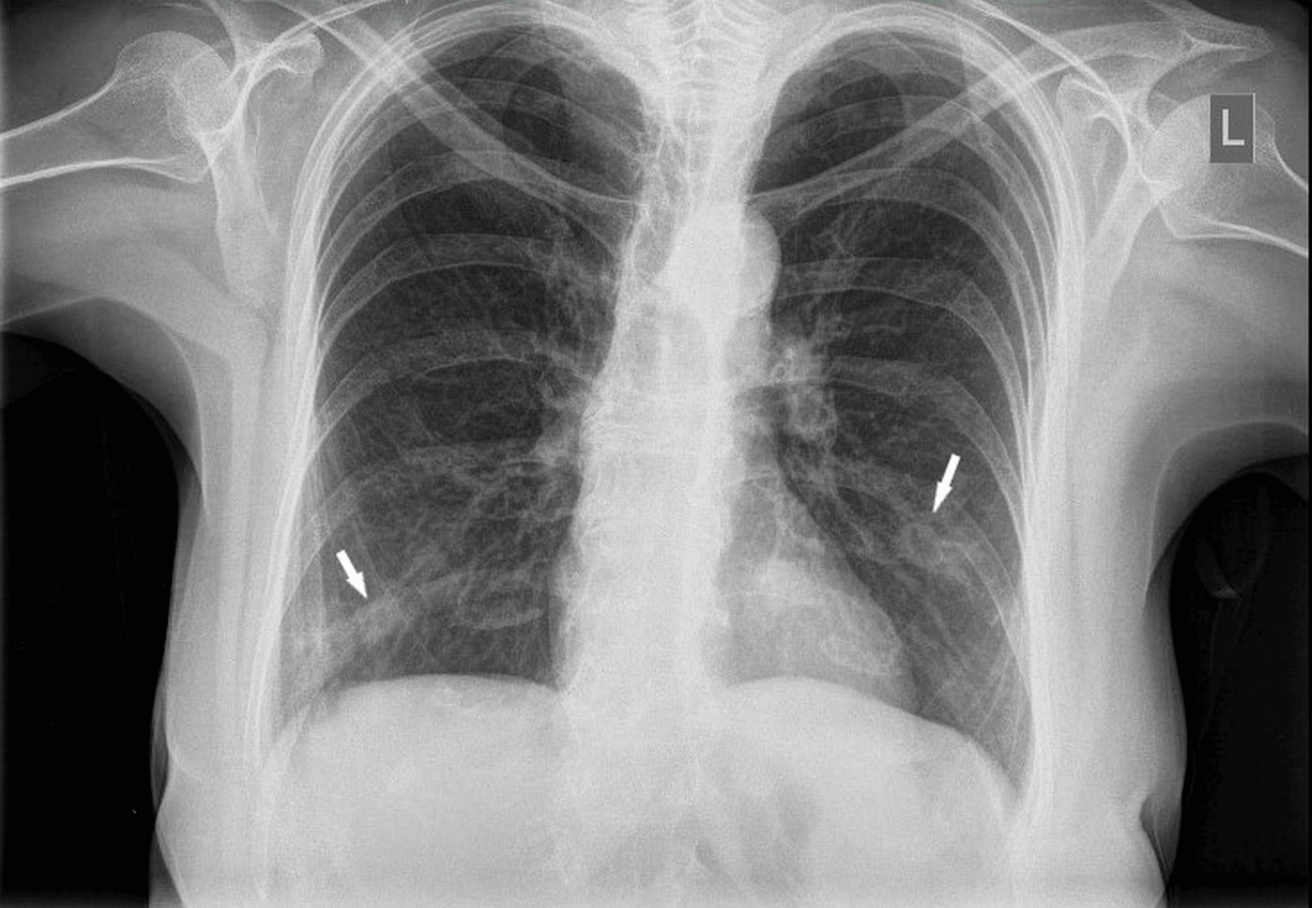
Posteroanterior chest radiograph showing bilateral cavitary pulmonary nodules (white arrows)
